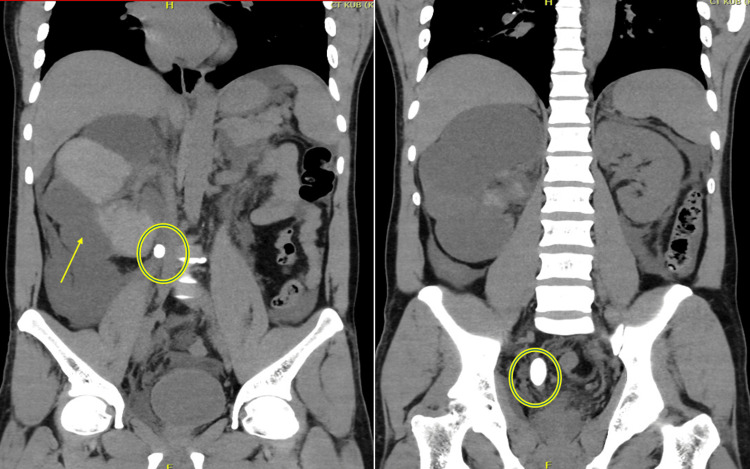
Coronal section of CT of the kidneys, ureters, and bladder (KUB). The arrowhead indicates severe right-sided hydroureteronephrosis and urinoma formation. The open circle highlights calculus in the upper ureter and the largest one, measuring about 1.8 cm.
